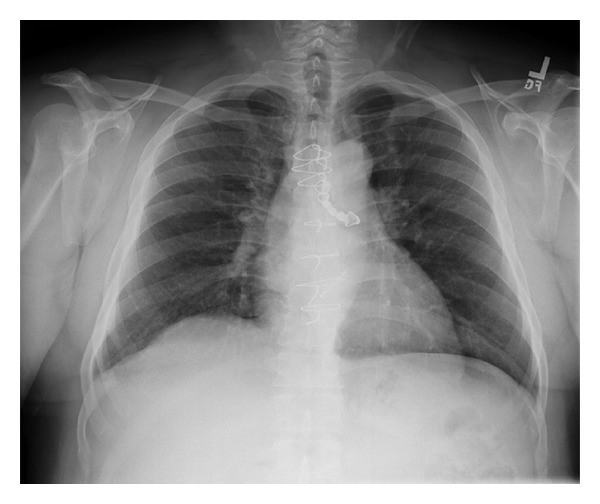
Chest radiograph shows a dental prosthesis lodged in the left mainstem bronchus.
